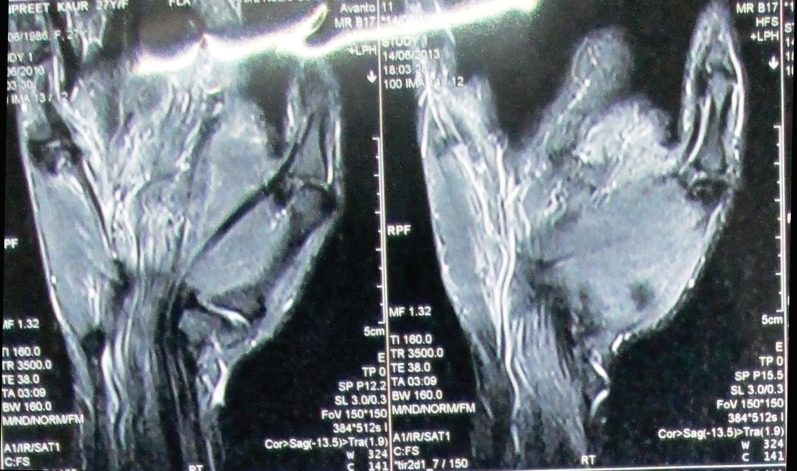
MRI picture shows the pathognomonic ‘cable-like’ pattern of the nerve
